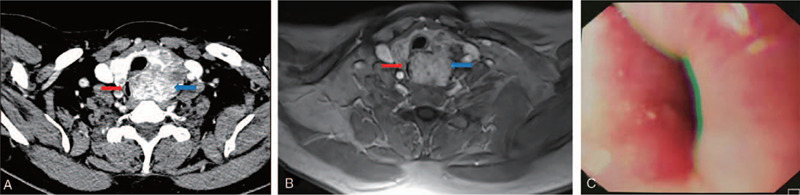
Initial examinations revealed a huge left thyroid mass with esophageal stenosis and invasion (the red arrow indicates the esophagus, and the blue arrow indicates the tumor). (A) CT scan. (B) MRI scan. (C) Esophagogastroduodenoscopy. CT = computed tomography, MRI = magnetic resonance imaging.
